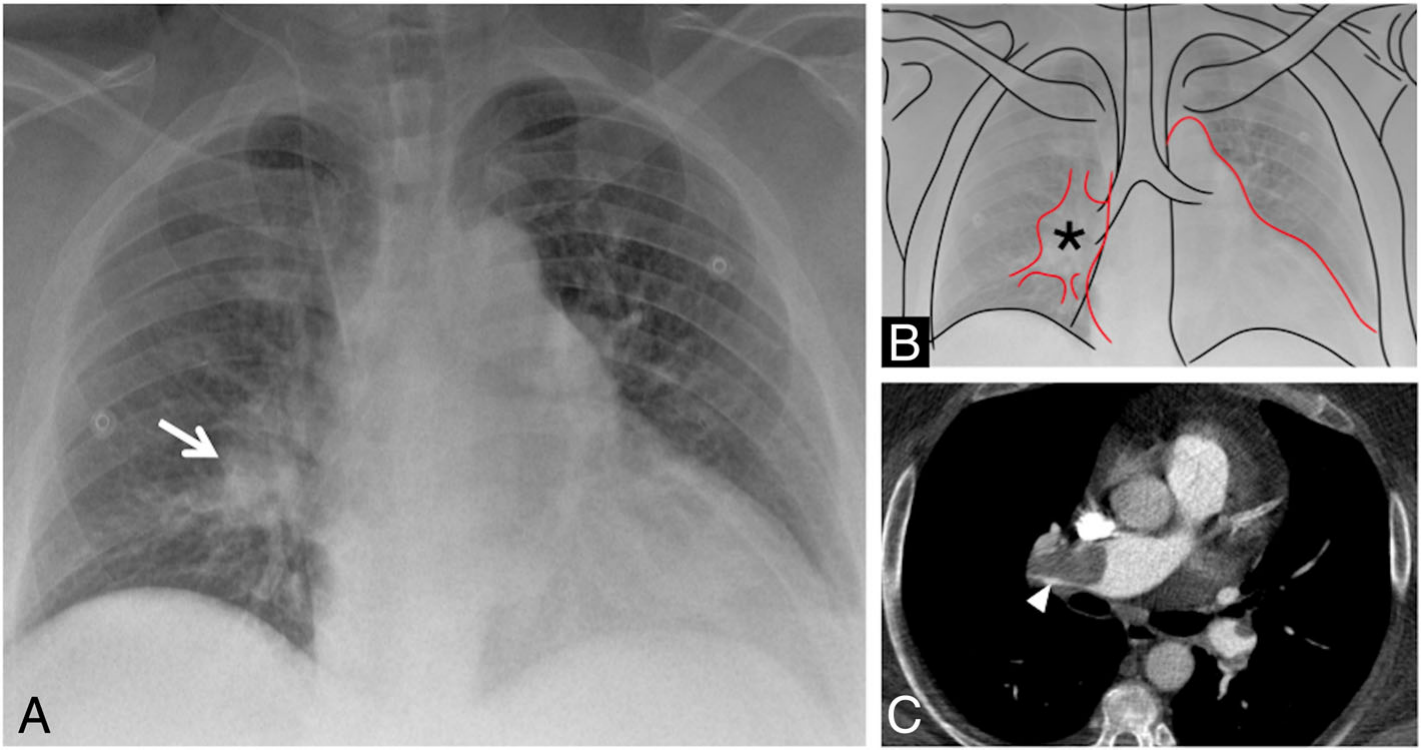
Fleischner sign. The “Fleischner sign” consists of a prominent central pulmonary artery upstream of cutoff (white arrows in a, black asterisk in b). It is frequently caused by a large embolus into the central pulmonary artery, as well depicted on axial CT image of the same patient (c, white arrowhead)
